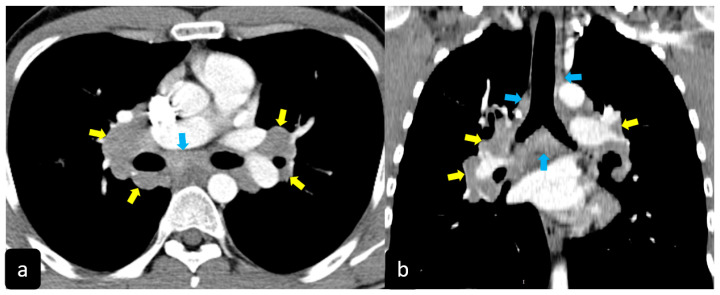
Axial (a) and coronal (b) CT scans in a patient with pulmonary sarcoidosis show the typical characteristic hilar ((yellow arrows) and mediastinal (blue arrows) bilateral lymphadenopathy.
